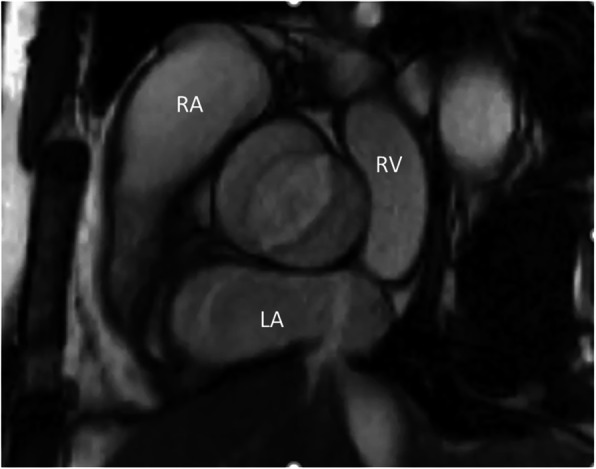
Bicuspid valve. Balanced steady-state, free precession cine CMR in the short-axis plane demonstrating a bicuspid aortic valve in a young woman with Turner syndrome. Echocardiography is first-line modality for assessment of cardiac valves. However, CMR can corroborate the valve morphology in case of suboptimal image quality with echocardiography. RA, right atrium; LA, left atrium; RV, right ventricle
